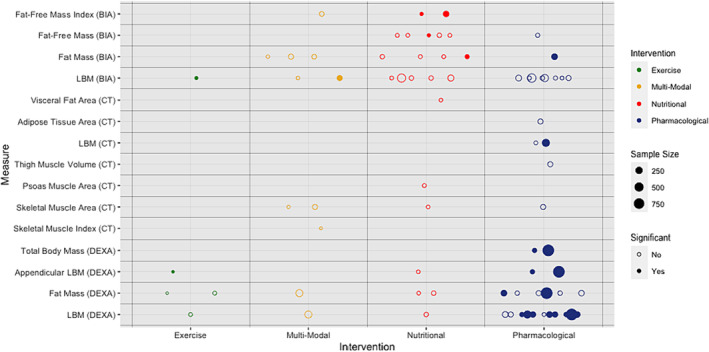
Summary of bioelectrical/radiological measures of body composition by intervention modality. BIA, bioimpedance analysis; CT, computed tomography; DEXA, dual‐energy X‐ray absorptiometry; LBM, lean body mass.
